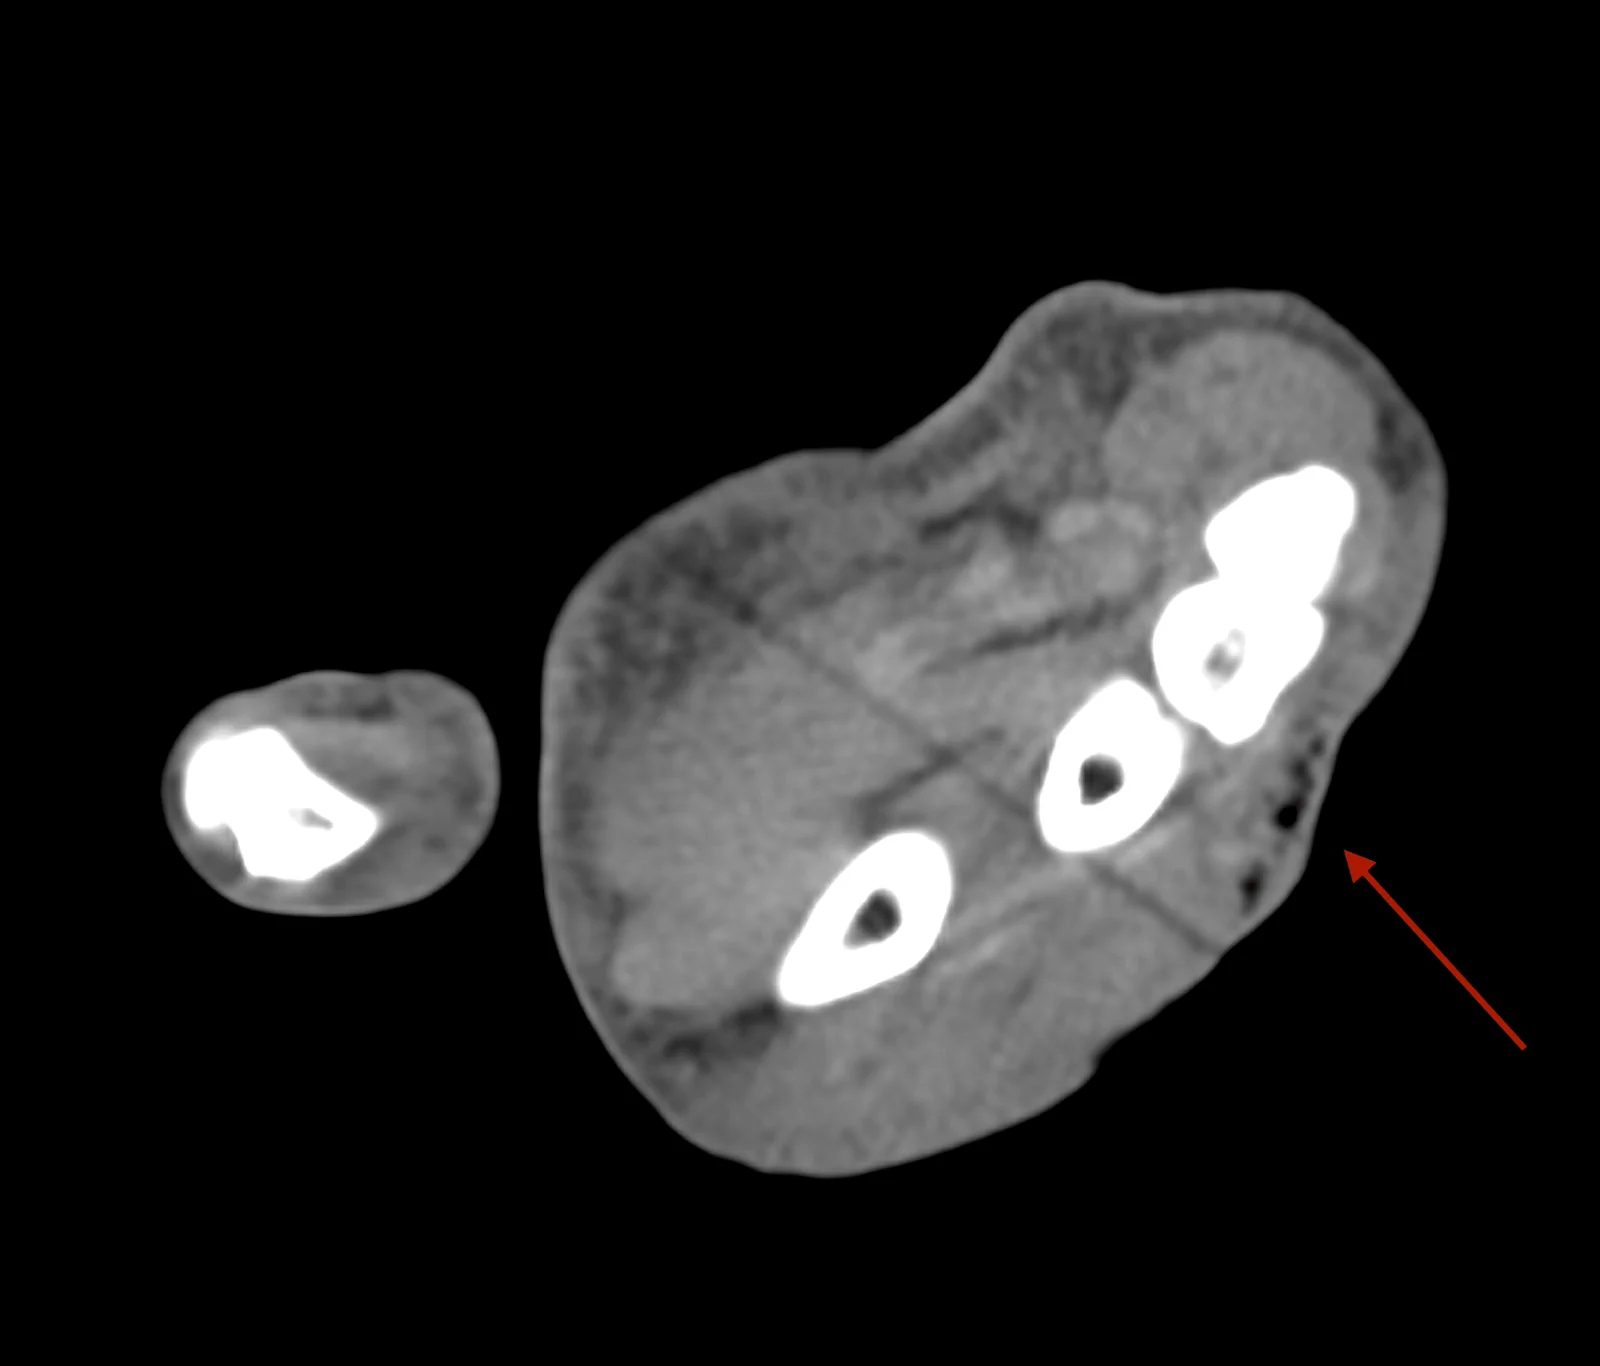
Transverse CT scan without contrast of the right hand. The red arrow points to emphysema over the dorsal aspect of the hand. CT=computed tomography
